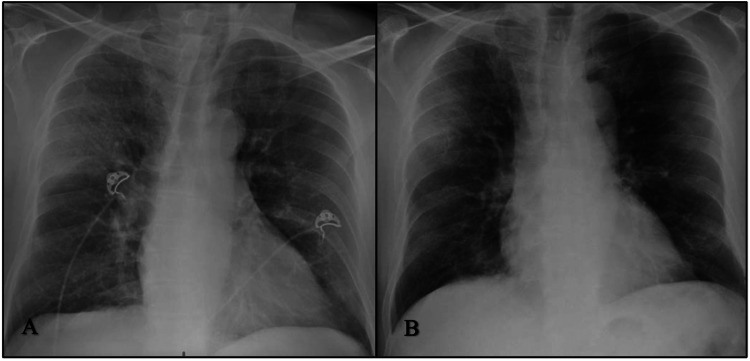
A: CXR from the admission day showing a right upper consolidation. B: CXR six days after admission showing improvement in interstitial thickening and airspace disease within the right lung. CXR: chest X-ray
